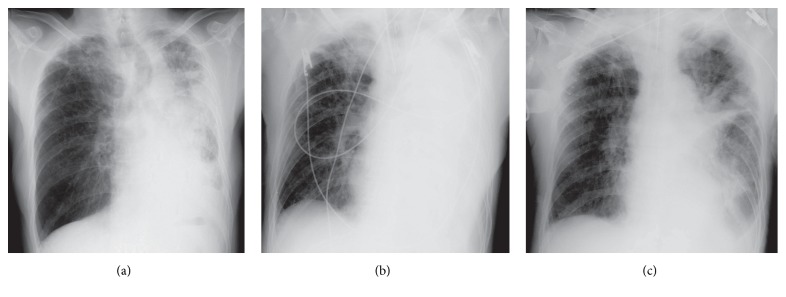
(a) Chest X-ray 3 days after admission revealed the progression of atelectasis in the left lung. (b) Chest X-ray on the day of intubation revealed complete atelectasis of the left lung. (c) Chest X-ray a day after the second electrocautery procedure showed marked improvement of the atelectasis of the left lung.
